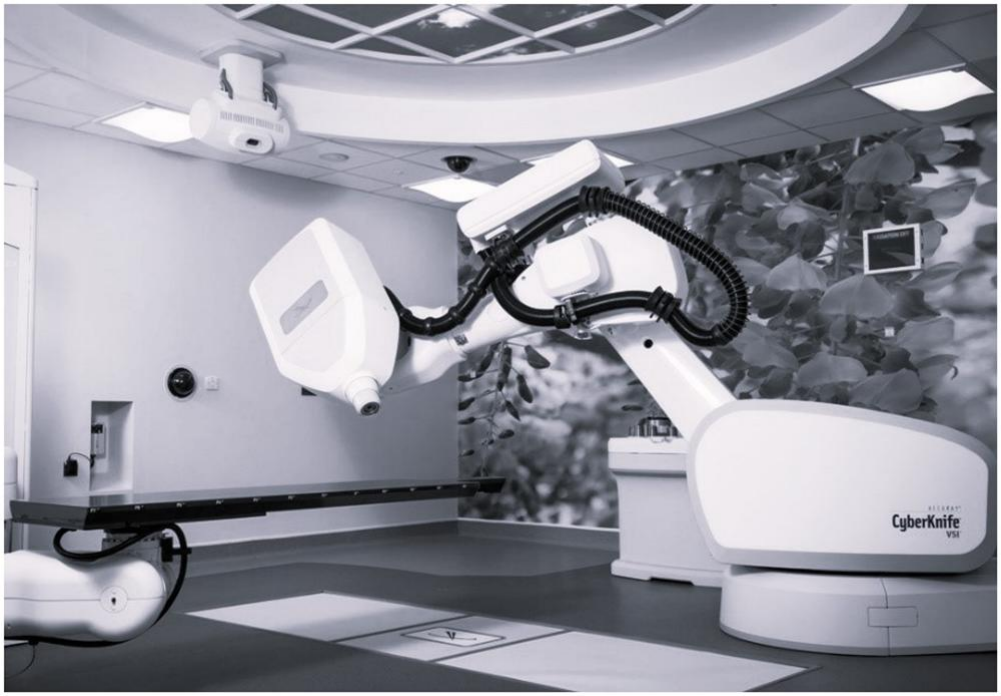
The CyberKnife system at Queen Elizabeth Hospital Birmingham. The linac and couch are each mounted on robotic arms with 6 degrees of freedom. The kV imaging system, consisting of 2 ceiling mounted X-ray tubes and 2 detector panels in the floor, detects patient movement. The CyberKnife system adjusts the position of the linac and couch to account for motion if necessary.
